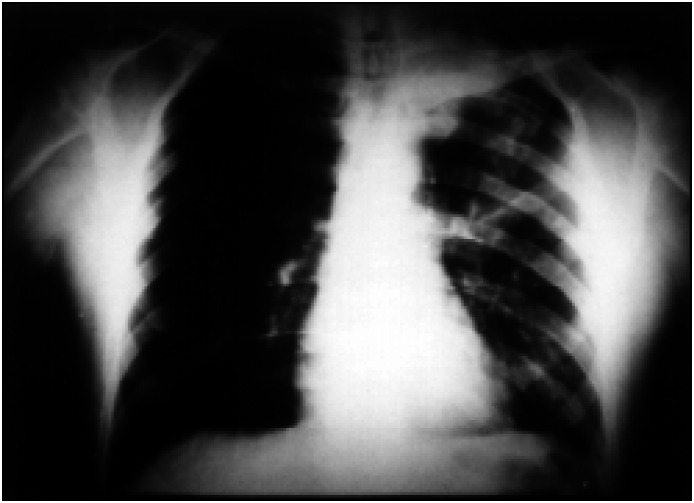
Chest X-ray revealing left upper lobe atelectasis.
